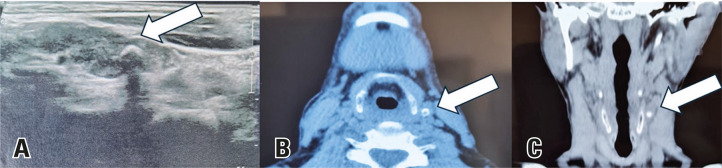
Target ultrasound (A) and computed tomography (B and C) showing an ectopic, non-descended inferior left parathyroid gland located in the submandibular region. The white arrows indicate the ectopic gland. Identification was facilitated by the observation of an intraparenchymal calcification
